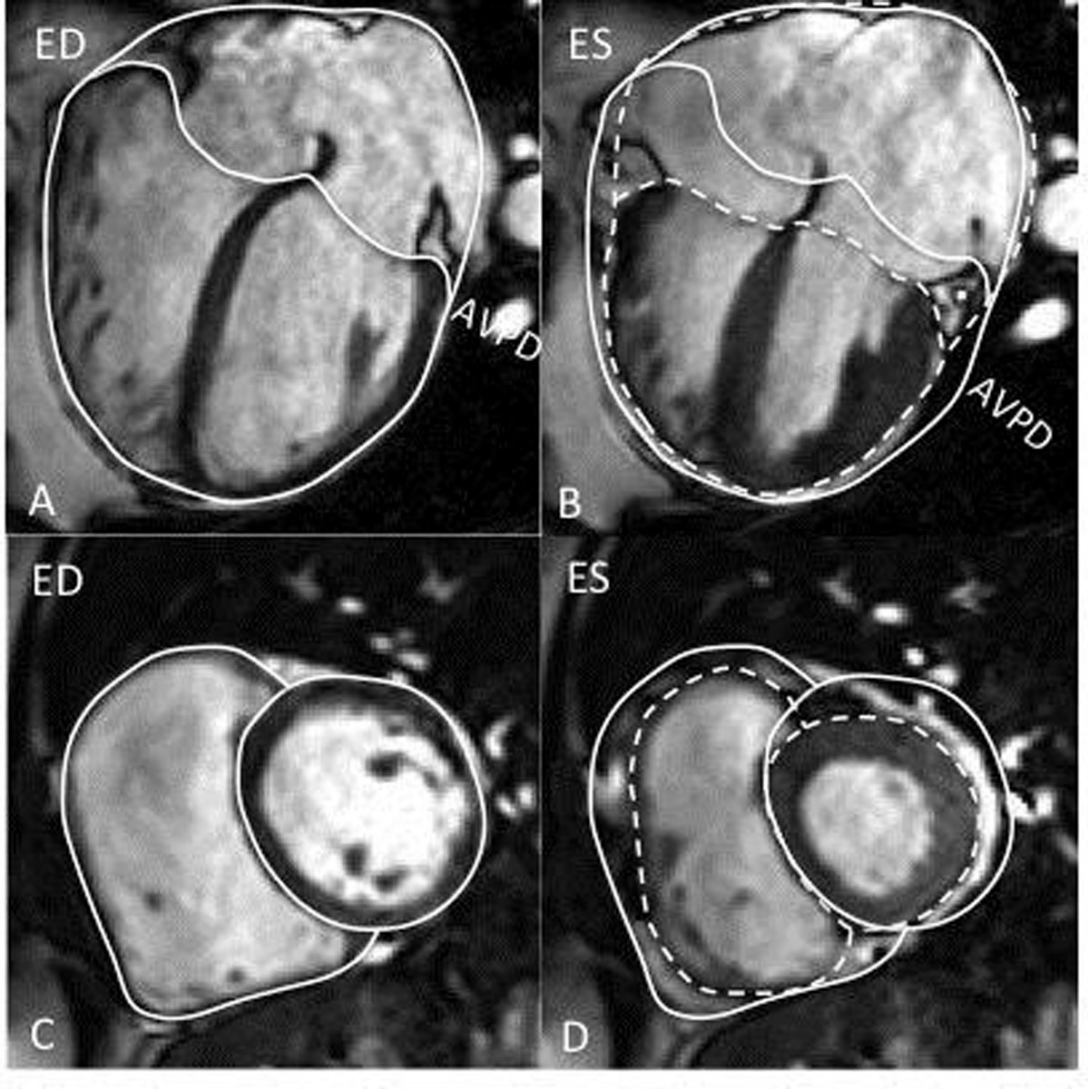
Cardiac MR images in the 4-chamber view (A and B) showing heart volume variations in a patient with ASD. The solid and the dotted white lines represent the heart contours in end-diastole (ED) and end-systole (ES), respectively. Atrioventricular plane displacement (AVPD) caused by longitudinal shortening of the ventricles is marked as a solid white line in ED and a dotted white line in ES.C and D: Short axis images of the same patient. The solid lines represent the epicardial contours of the LV and RV at ED and the dotted lines the epicardial contours at ES. This measurement was done in all short axis slices from the base to the apex. The difference in contours was used to calculate the radial contributions to LVSV and RVSV.
